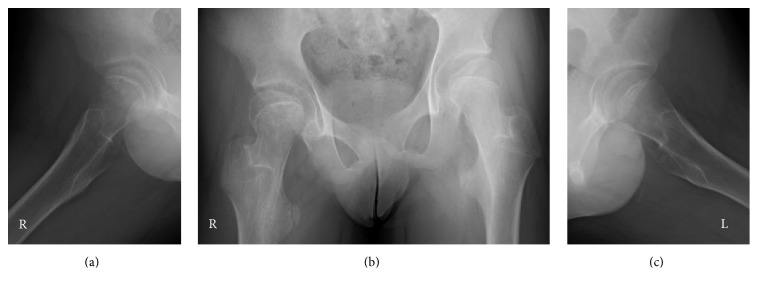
(a) Frog-lateral radiograph of the right hip joint showing almost normal. (b) Anteroposterior radiograph of the right hip joint showing lateral displacement of the right epiphysis and valgus neck shaft angles bilaterally. (c) Frog-lateral radiograph of left hip showing normal.
